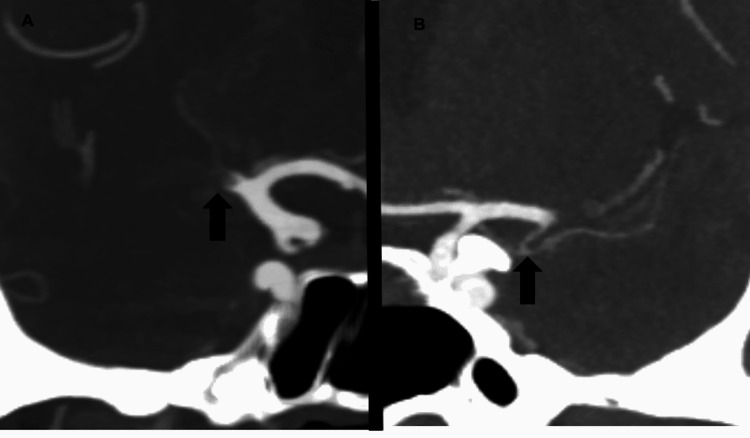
Coronal CT angiography example of the presence and absence of the anterior temporal artery (A) Right M1 proximal occlusion with no visualization of the anterior temporal artery. (B) Left M1 occlusion with patent anterior temporal artery.
